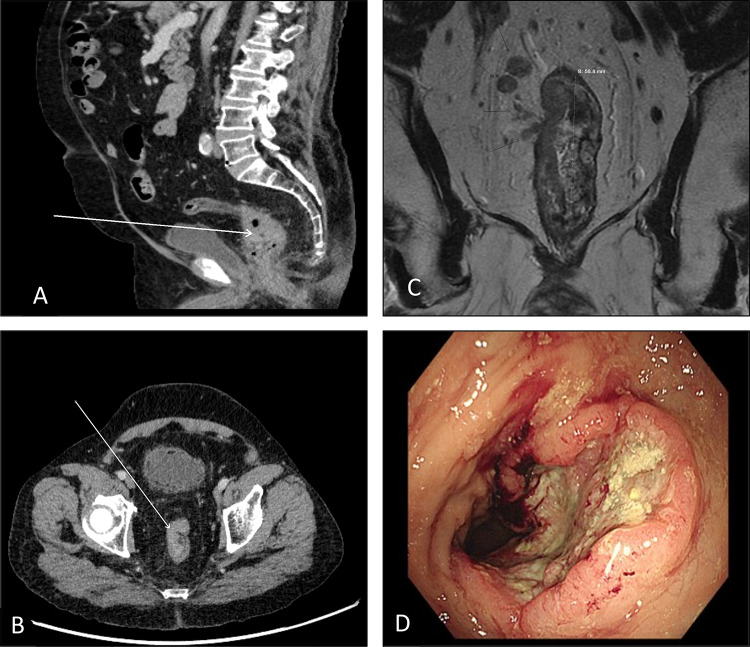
CTAP study demonstrating a mid-rectal lesion (white arrows A and B). C shows the subsequent T2-weighted coronal oblique small field of view MRI scan performed for local staging which demonstrates a 58 mm long tumor with further prognostic information including extramural vascular invasion (EMVI) and N1c nodal deposits (black arrows). The corresponding endoscopic image of the mid-rectal tumor is also included (D). This tumor was staged as T3d, N1c, EMVI positive, CRM involved, PSW negative, M0
